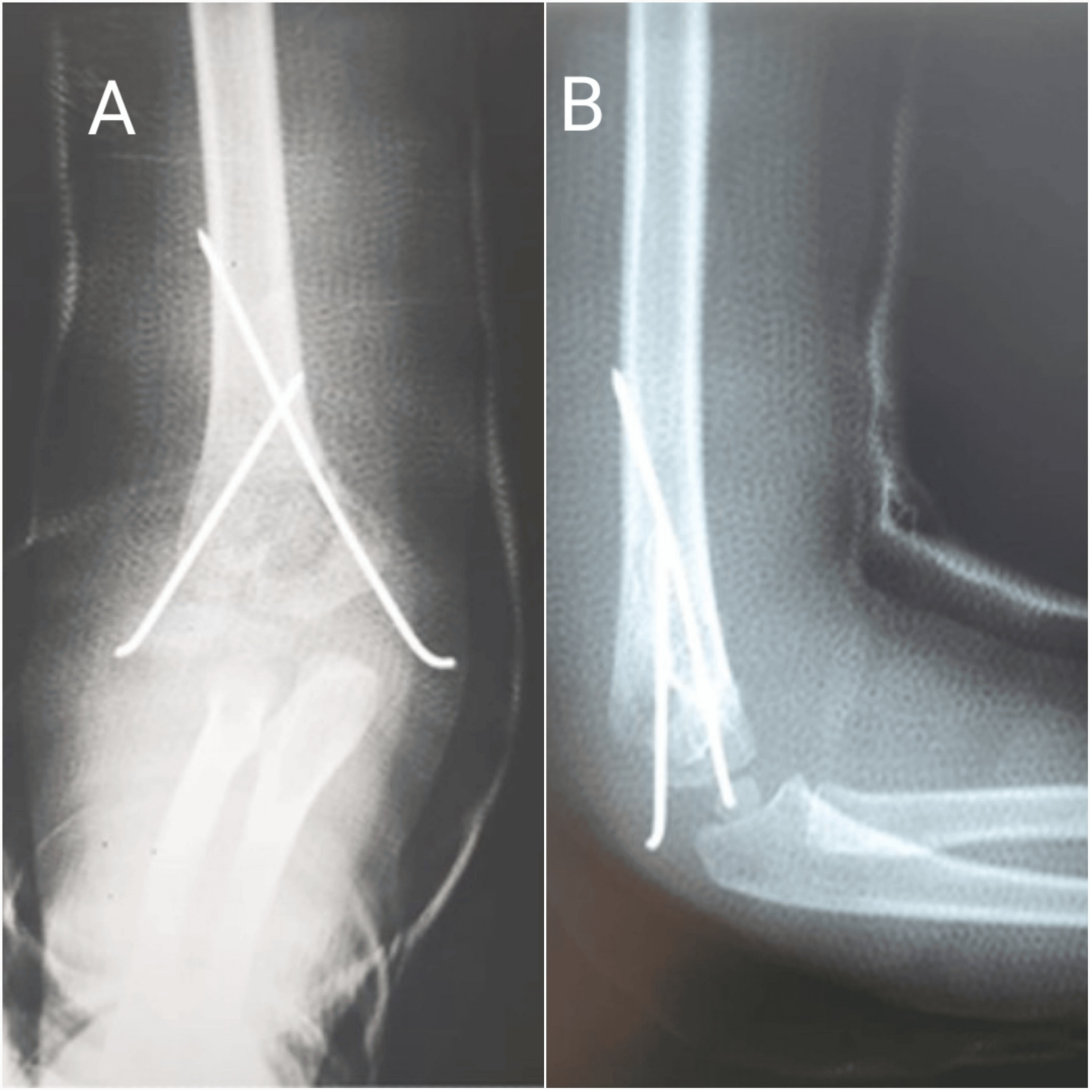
Post-operative X-rays of the right elbow demonstrating a satisfactory reduction following the fixation with two Kirschner wires A: Anterolateral view. B: Lateral view
